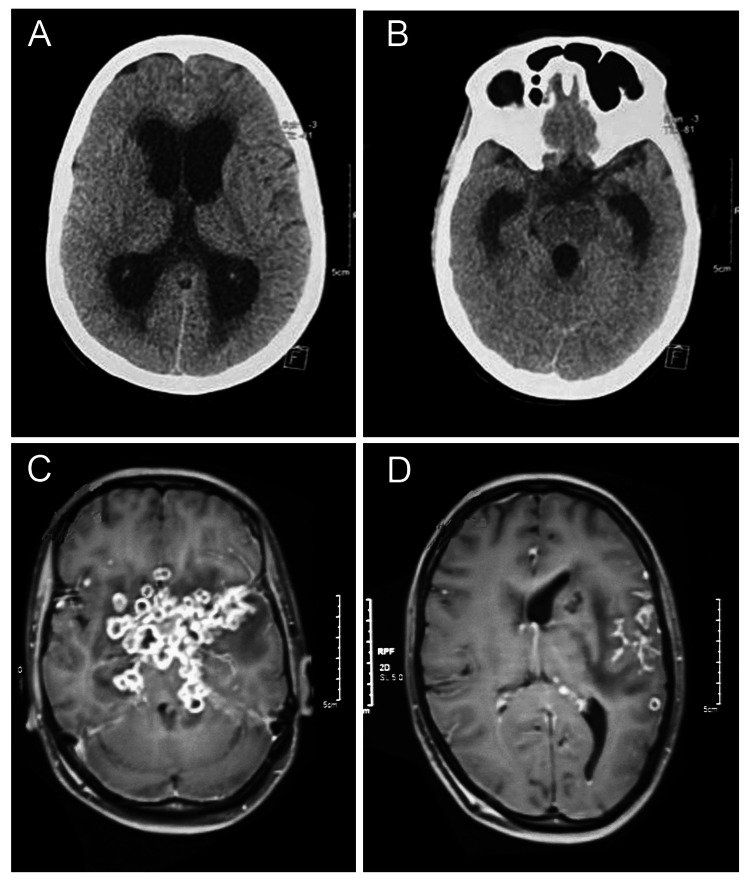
A. Non-contrast CT head axial section: dilatation of lateral and third ventricles suggestive of hydrocephalus; B. Non-contrast CT head axial section: dilatation of temporal horns of lateral ventricles and cerebral aqueduct; C. MRI brain gadolinium-enhanced T1 axial section: basal exudates with opto-chiasmatic arachnoiditis engulfing the base of the brain; D. MRI brain gadolinium-enhanced T1 axial section: leptomeningeal enhancement with thick exudates along the left Sylvian fissure
